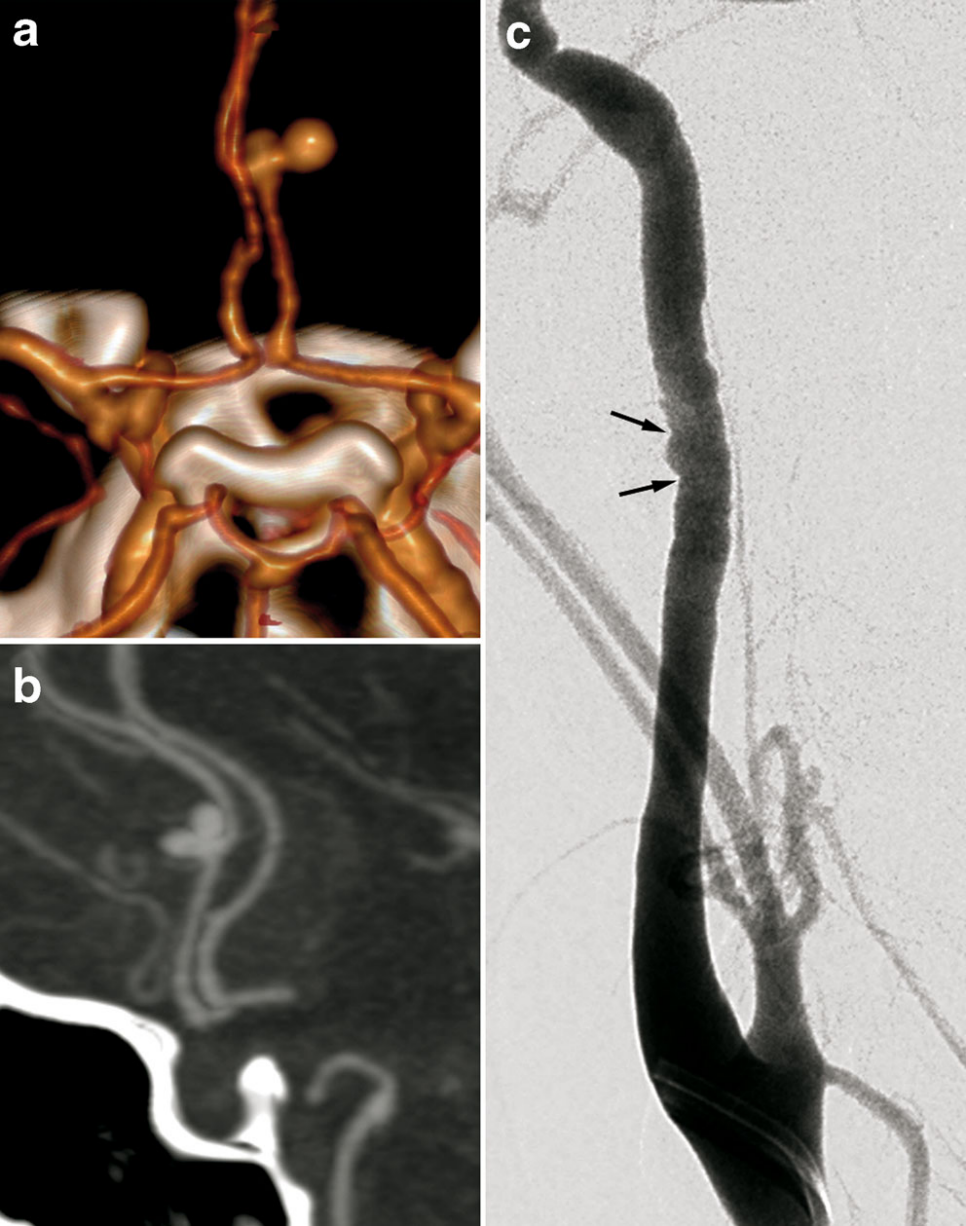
Radiologic findings in a 38-year-old female patient presenting with non-traumatic spontaneous subarachnoid haemorrhage. a Three-dimensional volume-rendered cerebral CTA showing a right peri-callosal artery aneurysm. b Cerebral CTA sagittal MIP reconstruction of the same aneurysm. c Therapeutic arteriography revealed typical right carotid artery medial FMD lesions (*black arrows*)
